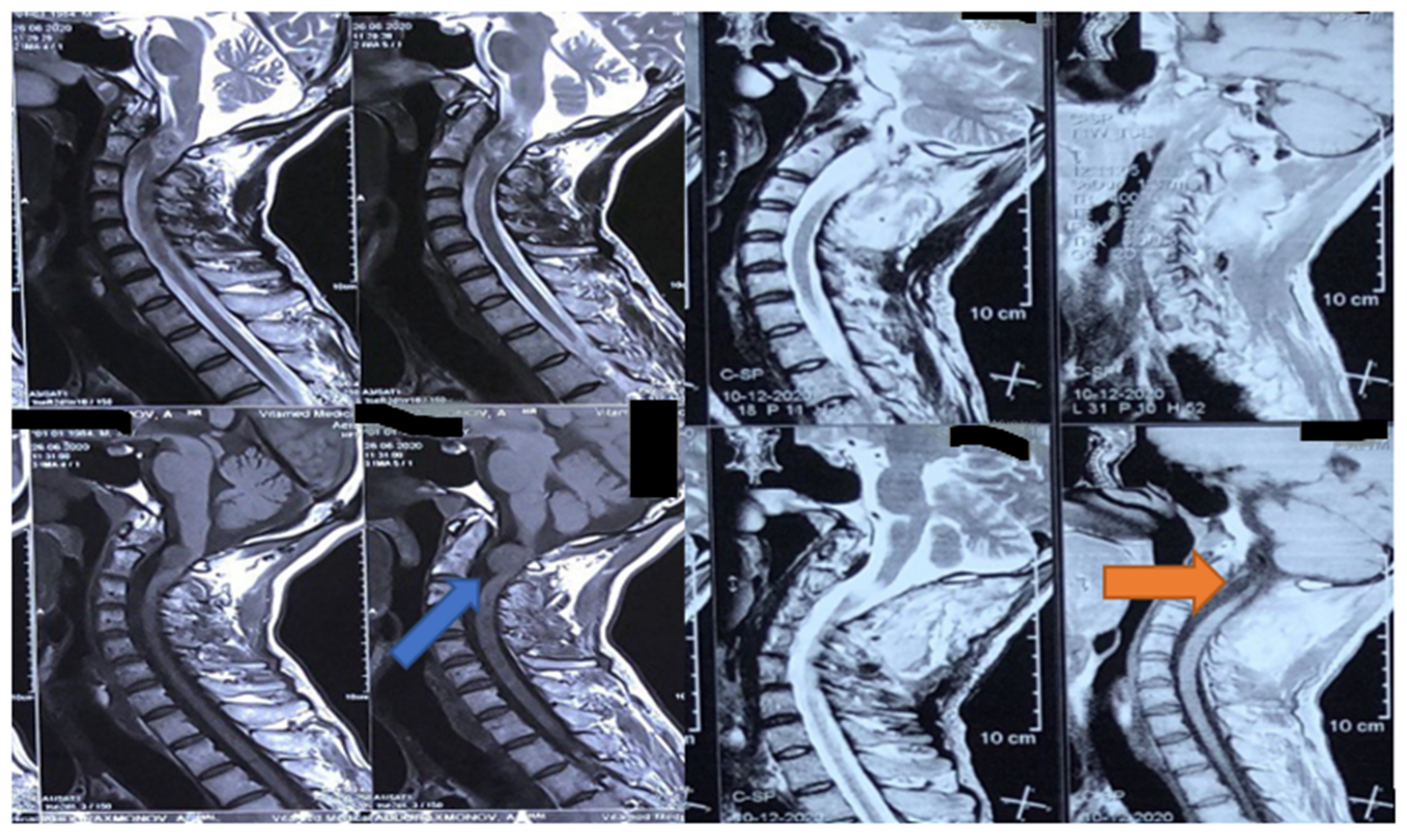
A pre-and post-surgery MRI of 32 years old with ependymoma of the roots of the cauda equina and syringomyelia between L3-L5 (blue arrow).
